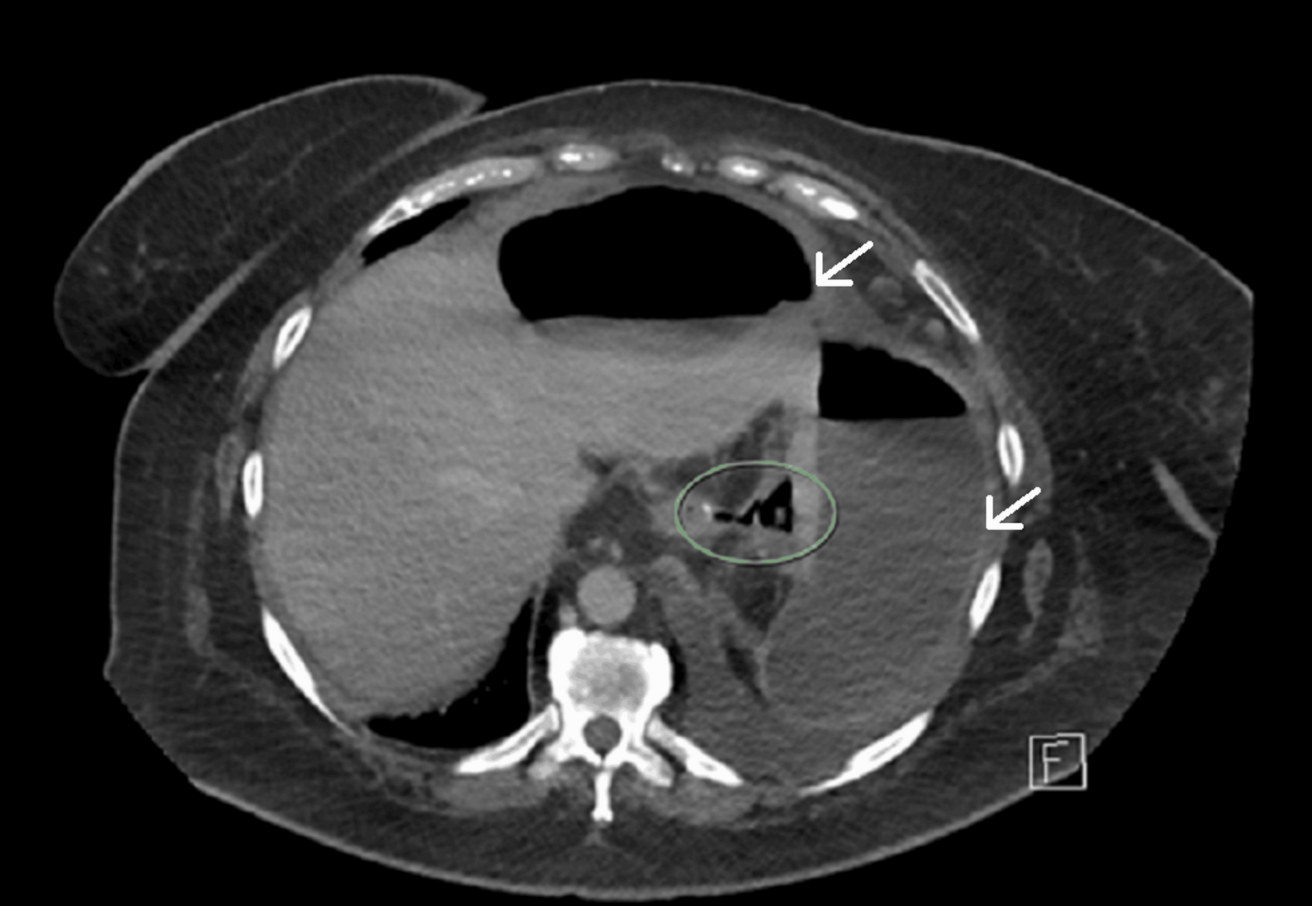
CT scan of intra-abdominal collection, day six post-splenectomy Green circle highlighting the source of gastric sleeve leak at the superior aspect of the staples line. White arrows indicate dumbbell-shaped intra-abdominal gas and fluid collection. CT, computed tomography
